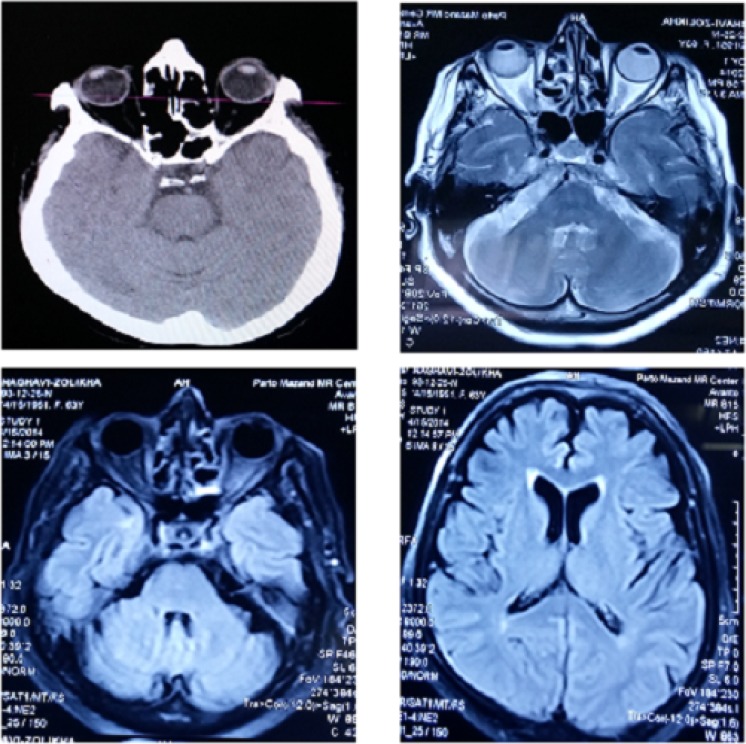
Brain computed tomography scan (top left image) and magnetic resonance imaging of patient.
